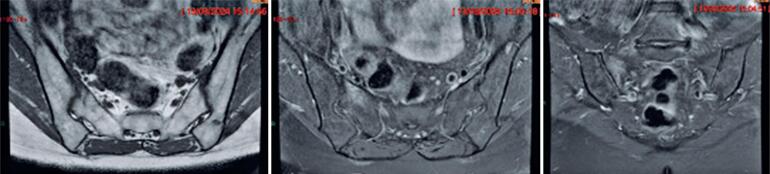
Axial T1, axial T2 with fat suppression, and coronal T2 with fat suppression MRI images showing an incomplete vertical fracture line without displacement on the anterior aspect of the right sacral ala, surrounded by mild bone edema, indicative of a subacute stress reaction
